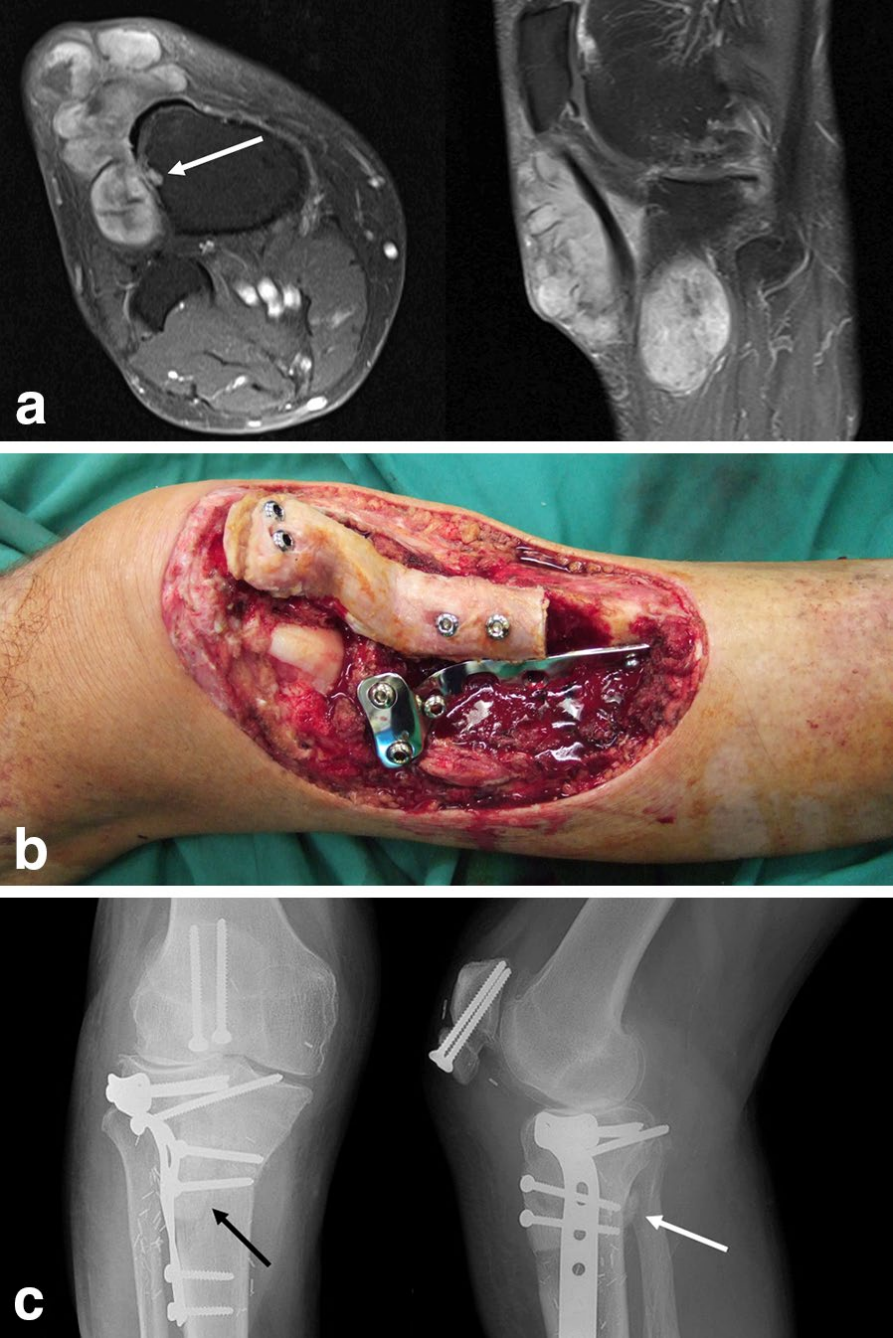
Radiologic studies and photographs of patient 4. a T1 gadolinium-enhanced magnetic resonance images of at the first presentation demonstrate partial tumor invasion into the lateral cortex of the proximal tibia (*arrow*). b After allograft reconstruction, the proximal tibia was reinforced with a lateral proximal tibial plate and screws. c Radiographs show a stress fracture in the proximal tibia 5 months post-operatively. A *black arrow* and *white arrow* point a thin oblique fracture line and posterior fracture-associated callus formation, respectively
